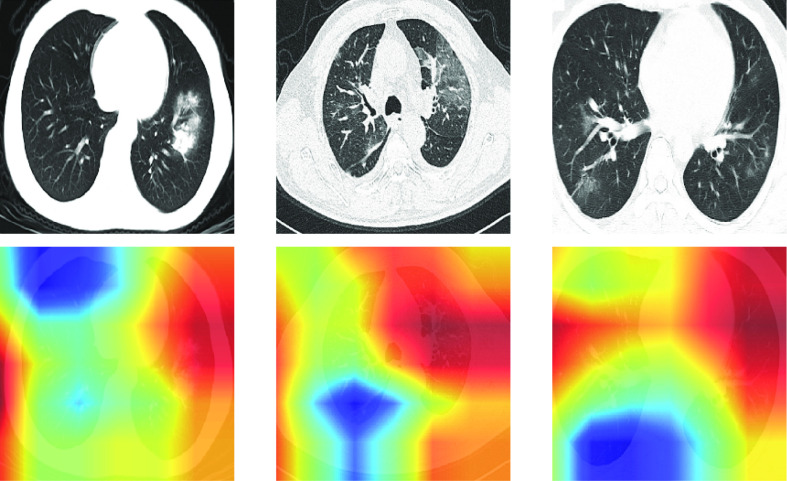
CAMs of some testing images for the classification of COVID-19 vs. other CT images. Red areas show which parts of the image the algorithm believes to be useful in the diagnosis.
